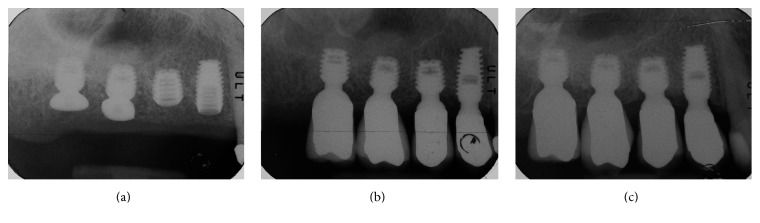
(a) Immediate postoperative radiography of premolar implants placed at the upper posterior maxilla (premolars); internal sinus lift cases were not included in this study. (b) Immediate X-ray obtained at crown insertion. (c) X-ray obtained at three-year follow-up showing the implant-restoration success.
